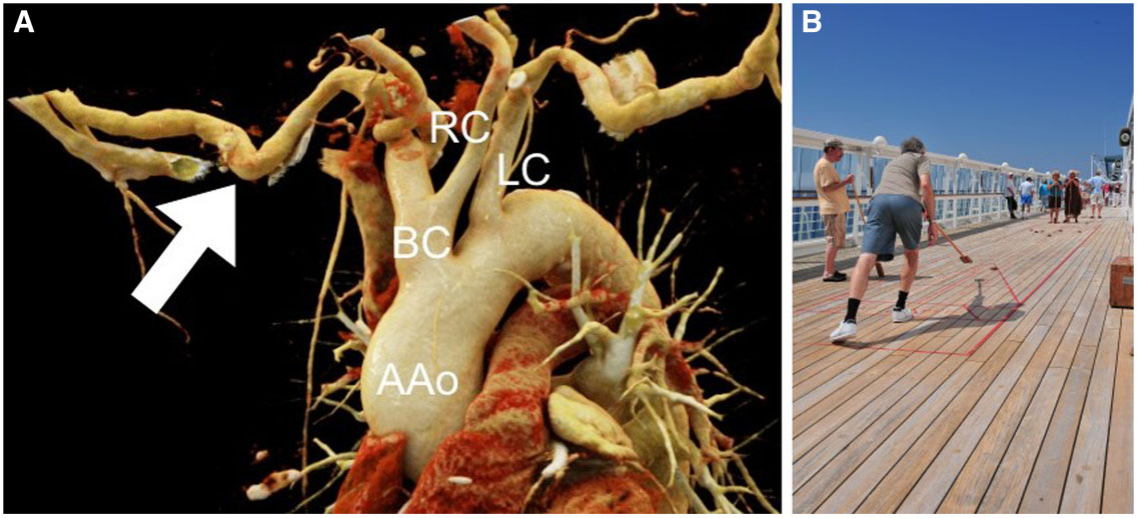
(A) 3D volume rendering of computed tomography. Ascending aorta, brachiocephalic artery, left common carotid artery, right common carotid artery, rectangular kinked axillary artery (arrow). (B) Shuffleboard on a cruise ship.
